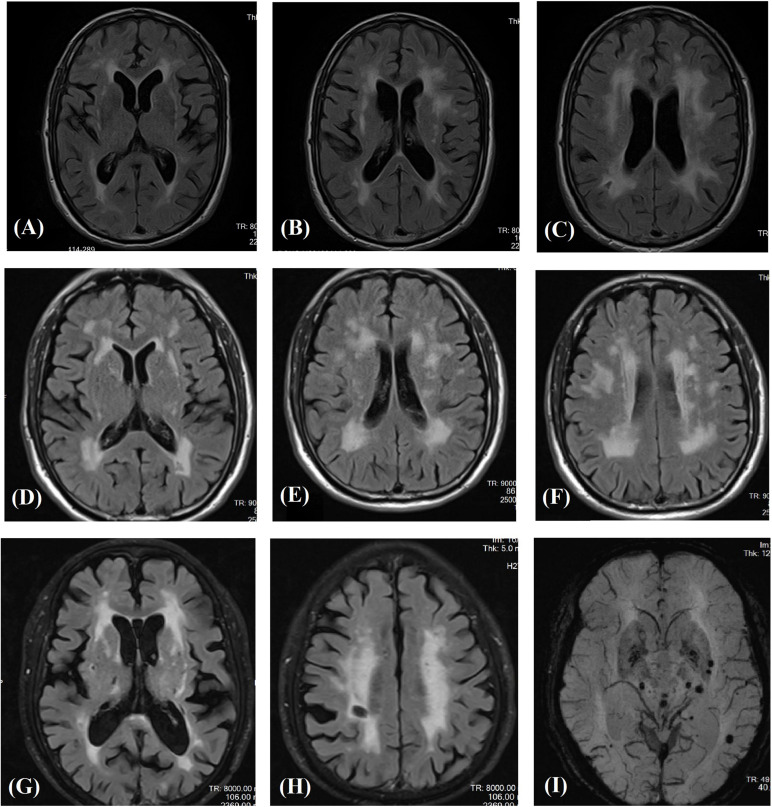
Magnetic resonance images in three patients carrying novel *NOTCH3* variants outside EGFr coding region. Fluid-attenuated inversion recovery images of the carrier of S2203Y (A–C), V1922L (D–F), and R1761H (G,H) showing multiple subcortical lacunar infarcts and characteristic diffuse leukoaraiosis with involvement of the external capsule. SWI images showed multiple microbleeds in basal ganglion and subcortical region of the carrier of R1761H (I).
